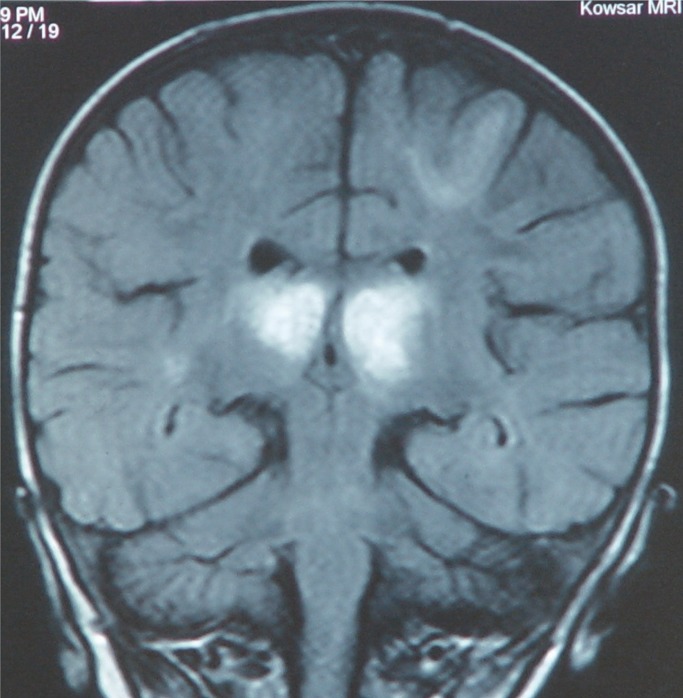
The coronal view of brain MRI with bilateral signal intensity in both thalamus
